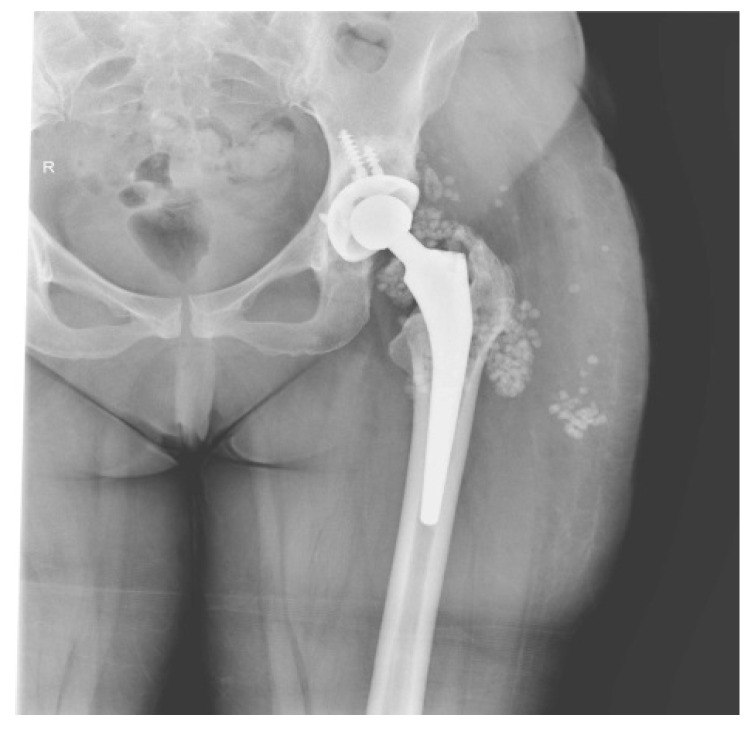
X-ray of the left pelvis—highlighting of Stimulan pearls around the prosthesis chamber and in the layer adjacent to the skin.
